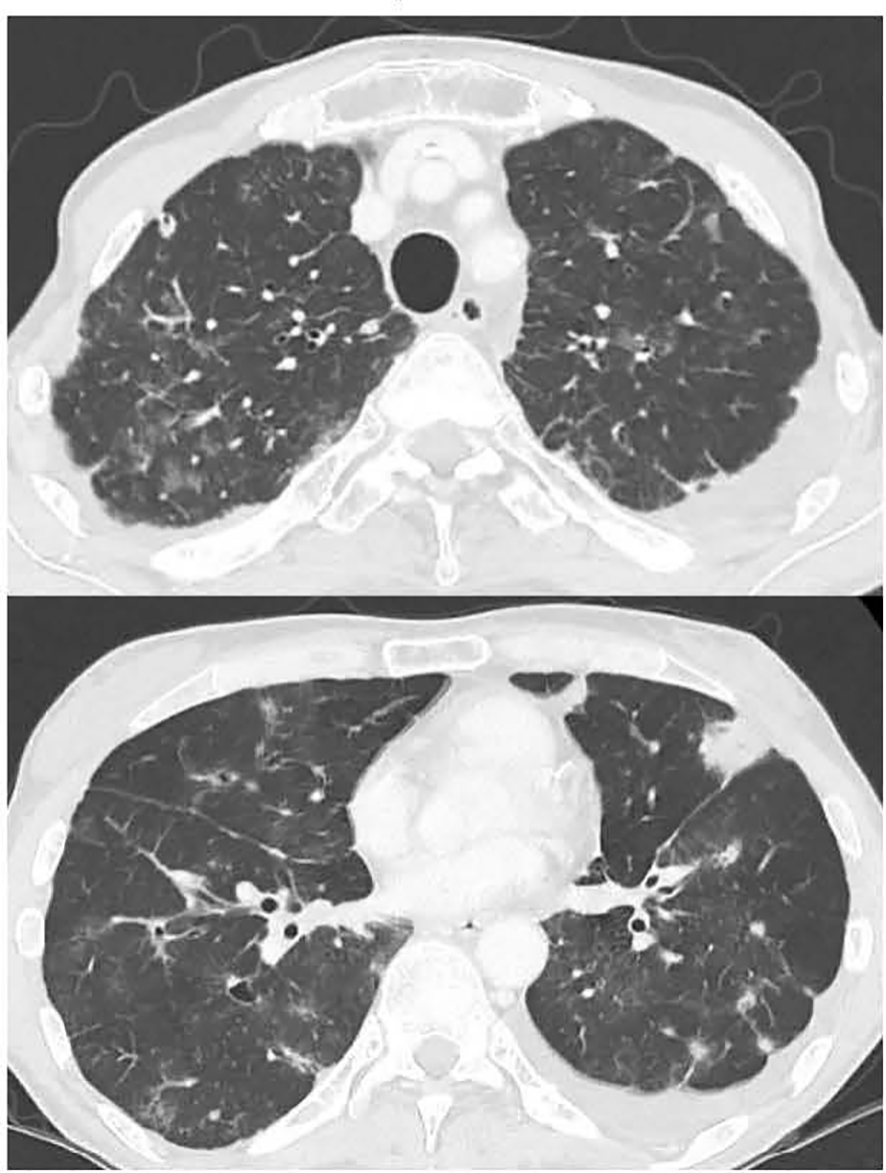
Chest CT showing the bilateral lung interstitial infiltrations.
